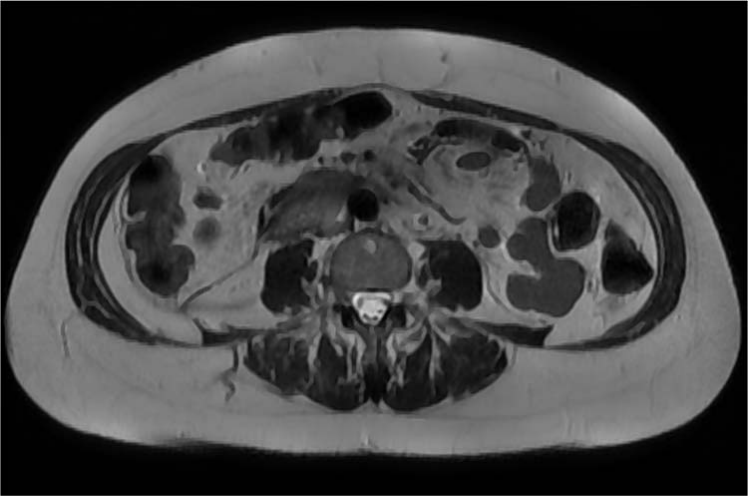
MRI abdomen, axial view. Resolving paraduodenal hematoma.
